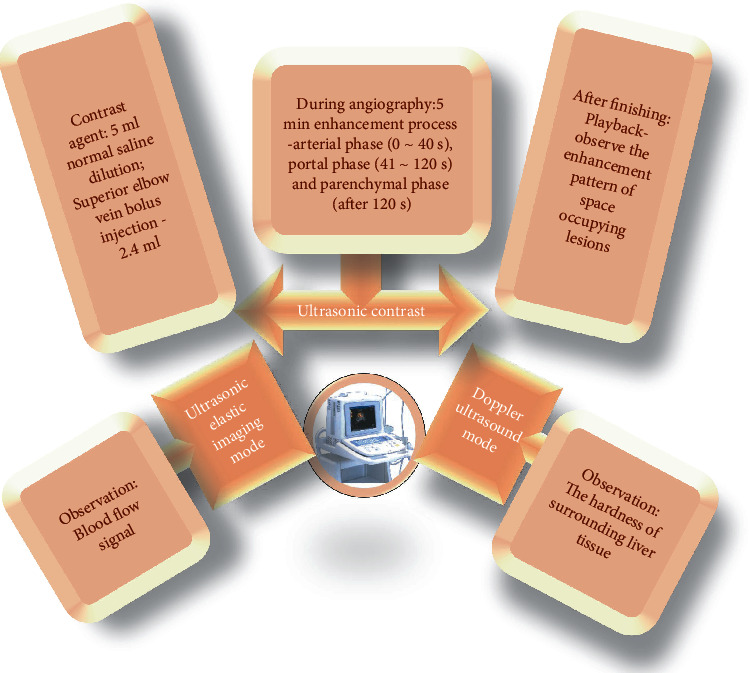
Ultrasonic examination methods.
